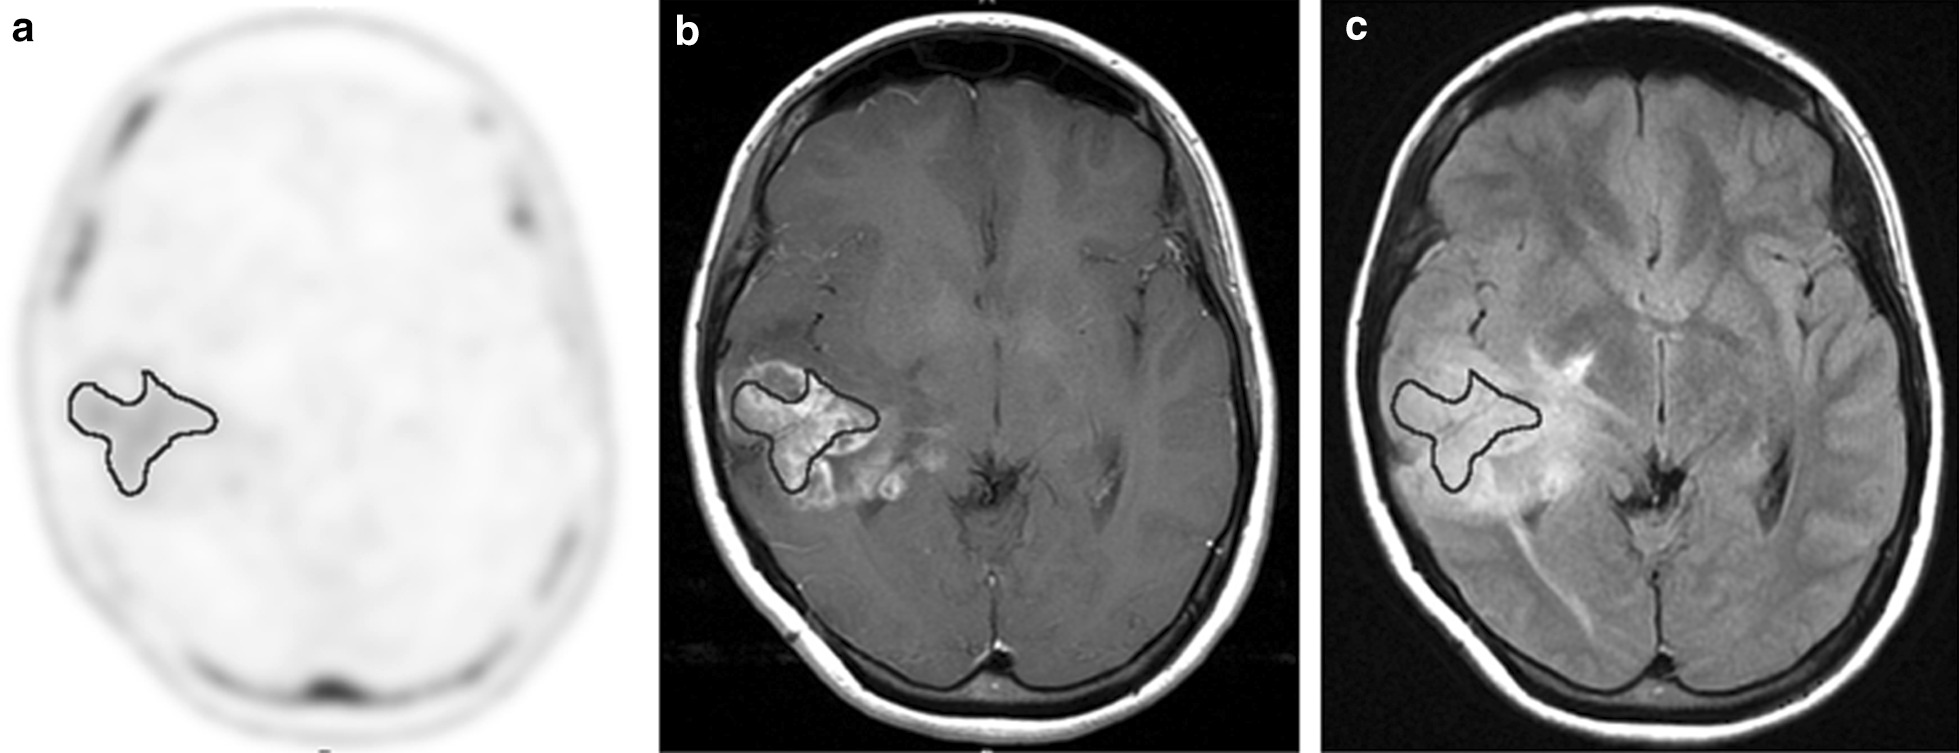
Radiologic images of a 29-year-old female with newly diagnosed glioblastoma. Transverse 4DST PET image a shows inhomogeneous uptake in the right temporal lobe (SUVmax = 1.87, T/N ratio = 5.84, PTV = 25.46 cm^3^). Transverse T1-weighted MRI with Gd enhancement b shows inhomogeneously enhanced lesion in tumor (GdV = 35.84 cm^3^). Region of interest shows proliferation area drawn on 4DST PET image from a. The proliferation area on 4DST PET image and Gd enhancement area on MRI differed slightly. The hyperintense area identified on transverse FLAIR MRI c is larger than the proliferation area on 4DST PET image (FLAIRV = 196.16 cm^3^)
